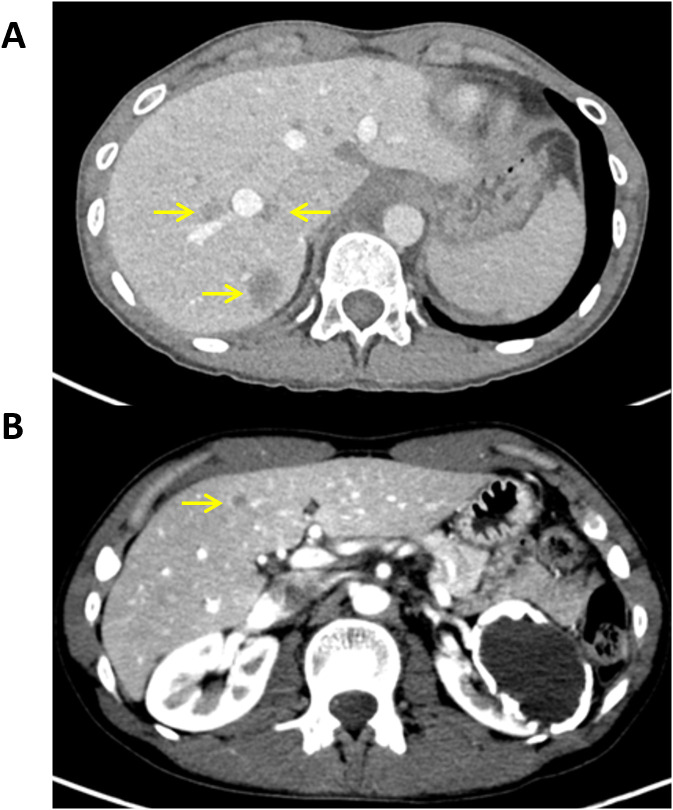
(A, B) Axial contrast-enhanced CT scans of liver before the second histotripsy treatment session displaying metastases throughout the right lobe of the liver (arrows).
